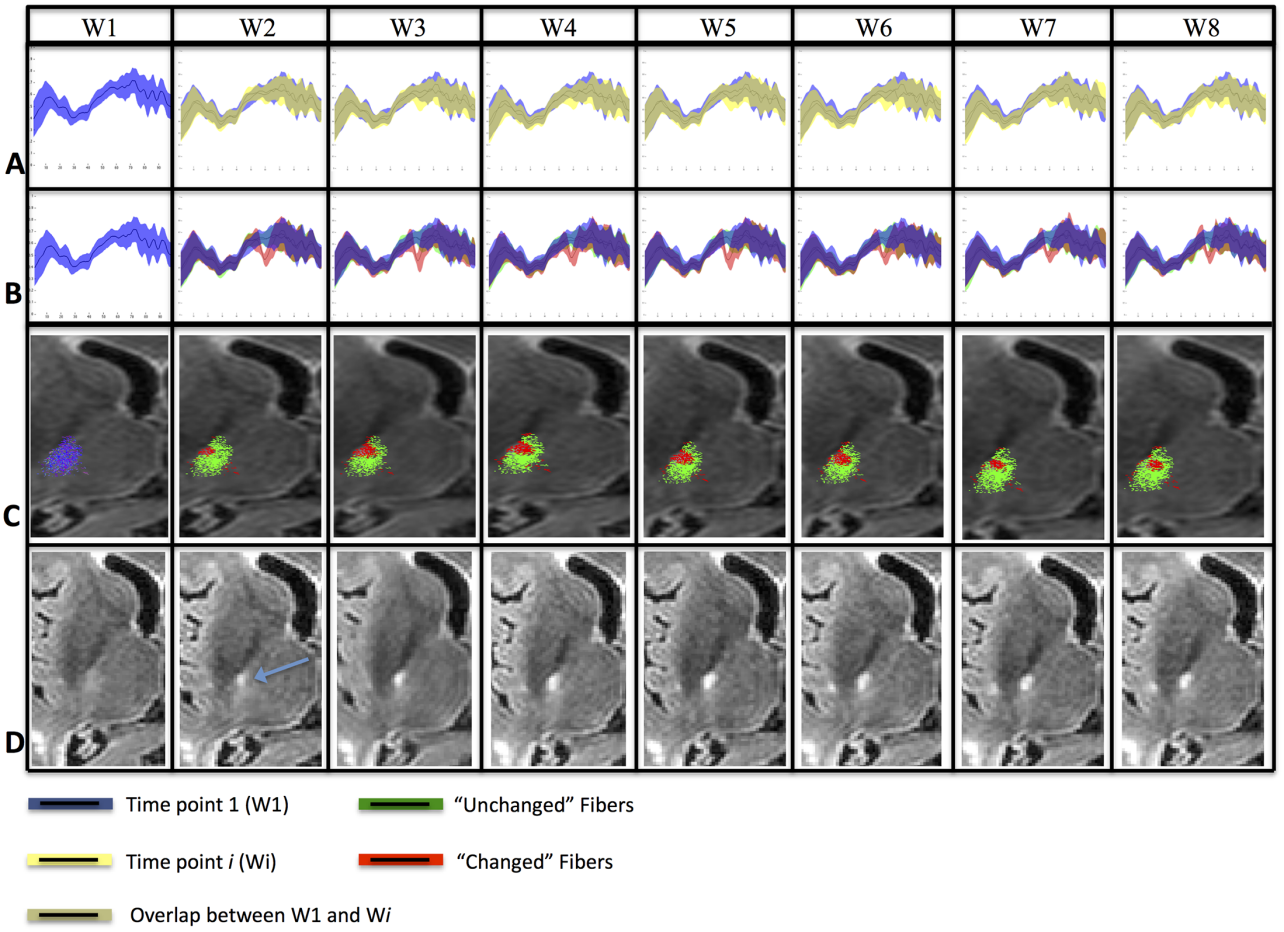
Longitudinal analyses of the FA values along the right CST of Patient1. (A) The “mean” method analysis showed no changes in the fiber-bundle between time-point 1 (W1, blue) and the other 7 time-points (yellow). (B) The “histogram” method analysis showed significant FA changes (red) between the reference time-point W1 (blue) and the others 7 time-points (W2 to W8) in different cross-sections of the fiber-bundle. (C) The “histogram” method allowed the distinction of “unchanged” fiber-subset (green) from “changed” fiber-subset (red) compared to the reference (W1) fiber-bundle (blue) as shown on the cross-sectional view of the CST. (D) FLAIR images of Patient1 showing the corresponding lesions.
